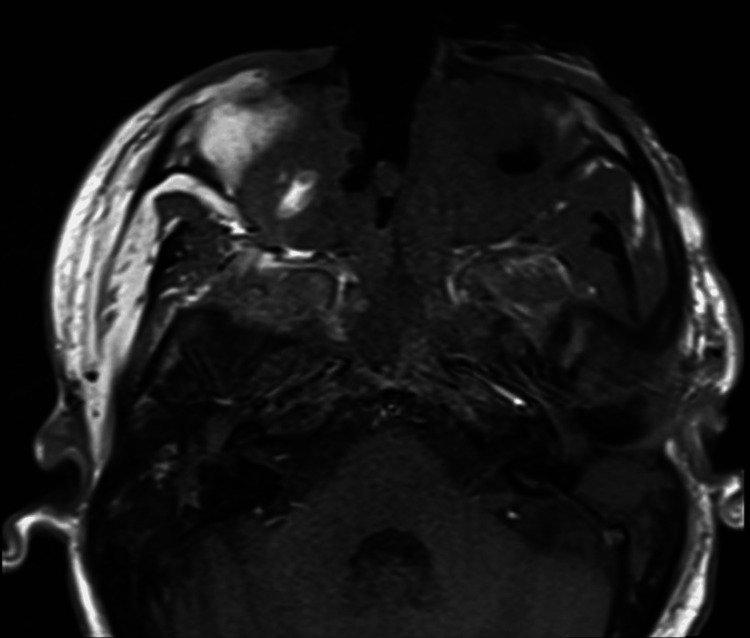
Axial T1-weighted MRI of the face and orbits two months into admission. Shown are stable orbital imaging and redemonstration of extensive deep, heterogeneously enhancing ulcerations of the face with osseous destruction of the facial bones and nasal cavity.
